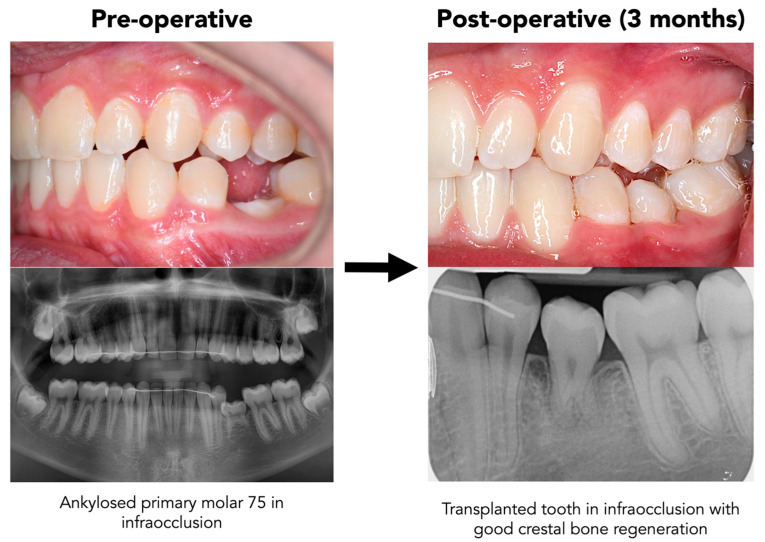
Clinical and radiographic comparison before and after autogenous transplantation of tooth 18 to site 75, demonstrating successful integration and preservation of periodontal structures.
